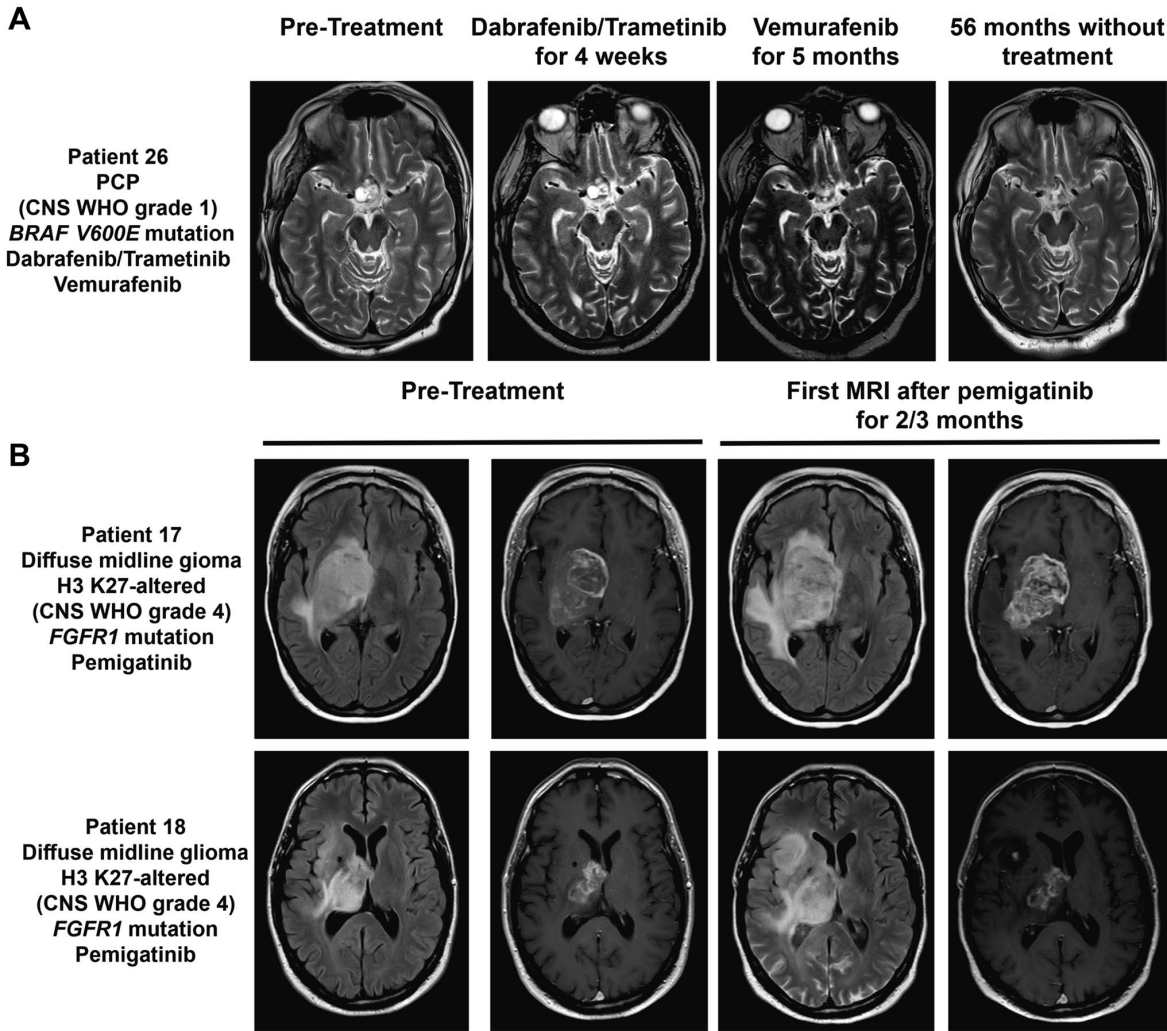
MRIs of selected cases. A axial T2-weightes magnetic resonance imaging sequences of patient 26 with a PCP with BRAF *V600E* mutation. First panel: after two tumor resections and one radiosurgery and before treatment with a BRAF inhibitor; second panel: after four weeks of treatment with dabrafenib/trametinib; third panel: after five months of treatment with vemurafenib (the patient was switched from dabrafenib/trametinib to vemurafenib due to side effects despite PR); fourth panel: after 56 months without therapy. B axial T2/fluid-attenuated inversion recovery-weighted magnetic resonance imaging sequences (left panel) and T1 post-contrast MRI (right panel) of two patients with recurrent H3K27-altered diffuse midline glioma and mutations in FGFR1 before and 2 months (patient 18)/ 3 months (patient 17) after start of treatment with pemigatinib
